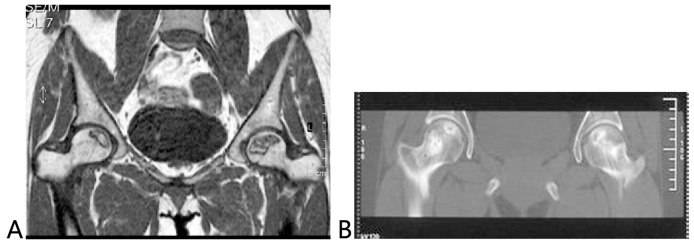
A 31-year-old patient with ONFH after SARS. A: Preoperative magnetic resonance image showed ARCO stage IIb/CJFH Type C (the right hip) and ARCO stage IIc/CJFH Type L1 ONFH (the left hip); B: This Computed tomography scan was performed at five years after the impacted bone grafting alone (without addition of BMP2) and showed the femoral head maintained its spherical shape an incomplete repair for the necrosis with the residual lesion at the subchondral bone of the femoral head.
